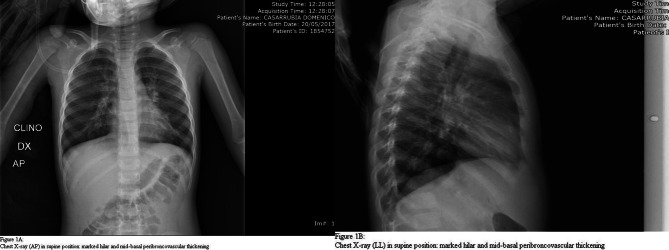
Chest X-ray (AP) in supine position. (A) Marked hilar and mid-basal peribroncovascular thickening. (B) Marked hilar and mid-basal peribroncovascular thickening
